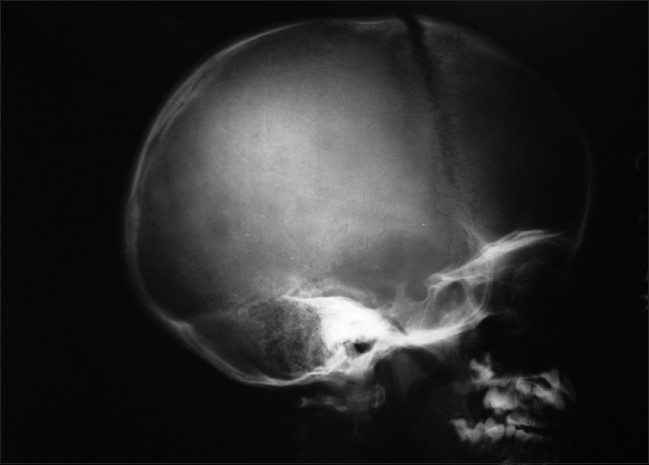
Lateral radiograph of skull vault showing an osteolytic lesion with erosion of inner and outer tables associated with sutural diastasis and periosteal reaction
